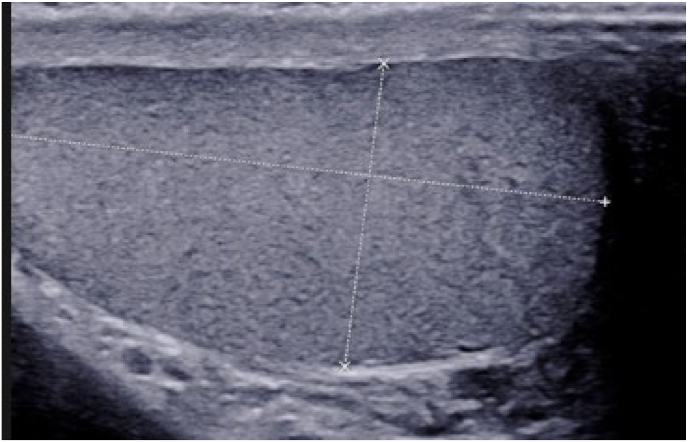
Testicular ultrasound showing a normal appearance of the testicles.
